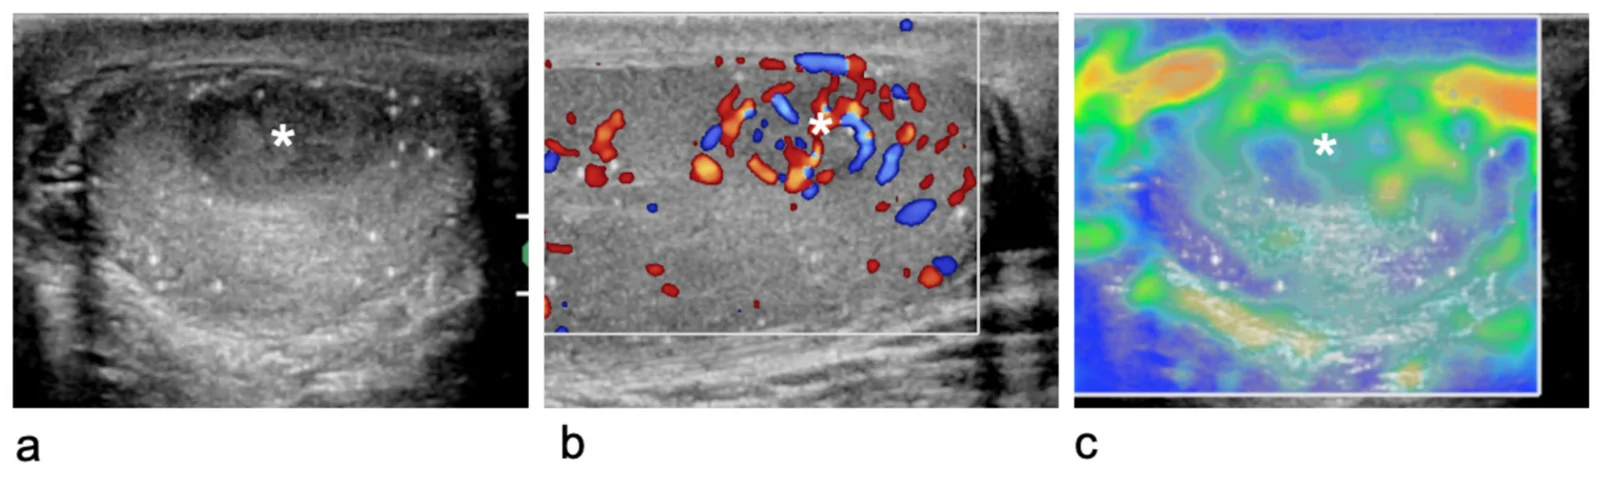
Small, non-palpable testicular lesion (asterisk) incidentally detected at ultrasound in a 25-year-old man investigated during fertility work-up. (a) Grey-scale ultrasound shows a heterogeneous left testis with microlithiasis and a hypoechoic lesion. The lesion is hypervascular on colour Doppler imaging (b) and predominantly soft on elastography (c). At surgery, the lesion proved to be a seminoma.
